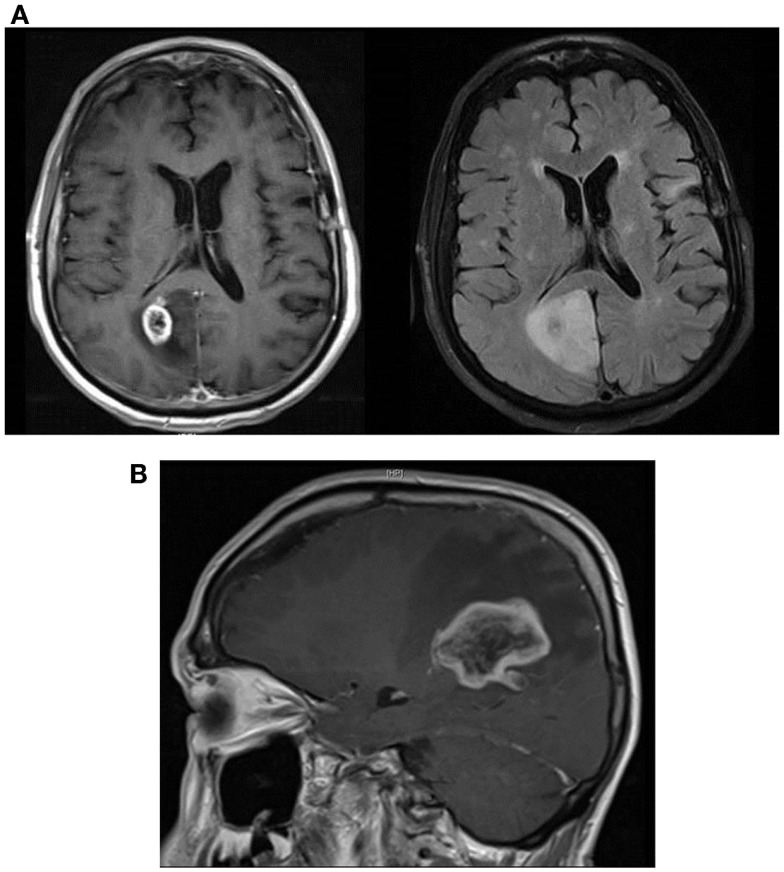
(A) Sagittal T1 post-contrast showing large area of right occipitoparietal lesion with a ring-like enhancing pattern (left image) and axial T2 FLAIR MRI showing substantial peri-lesional edema (right image). (B) Sagittal T1 post-contrast MRI showing marked increase in the size of the right parietal lobe lesion with surrounding local edema and enhancement.
